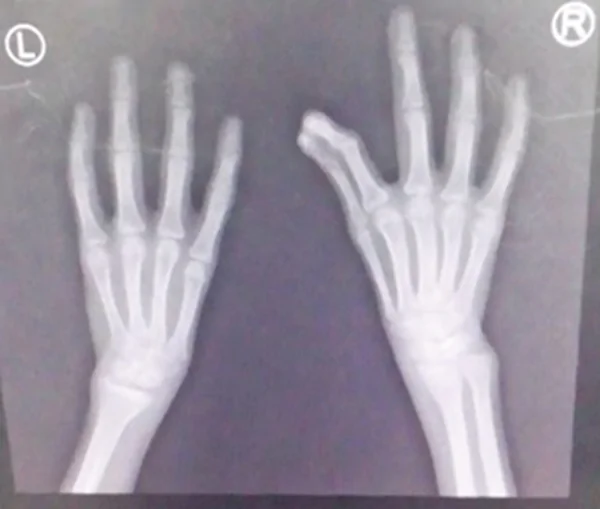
Skeletal radiograph of the upper limb showing absent left distal radial bone and absent first metacarpal and phalanges on the left side, with partially fused left carpal bones, hypoplastic right first digit with soft tissue syndactyly of the first and second digits.
